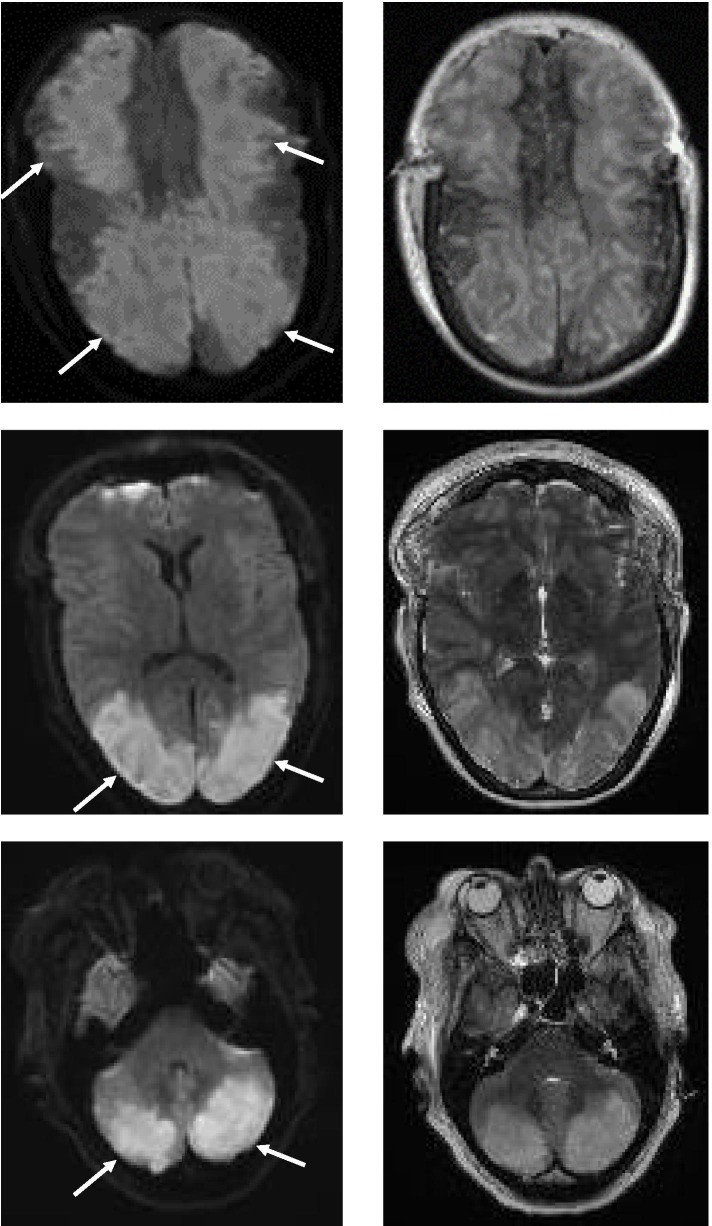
Brain MRI (transversal sequences) in a fatal case of PRES due to hypertension. Bilateral, symmetric and extensive diffusion restrictions (on diffusion-weighted imaging (DWI)) (*white arrows*) (left panels) and T2 hyperintense signal (right panels) due to full-blown manifestation of PRES with following bifrontal craniotomy
